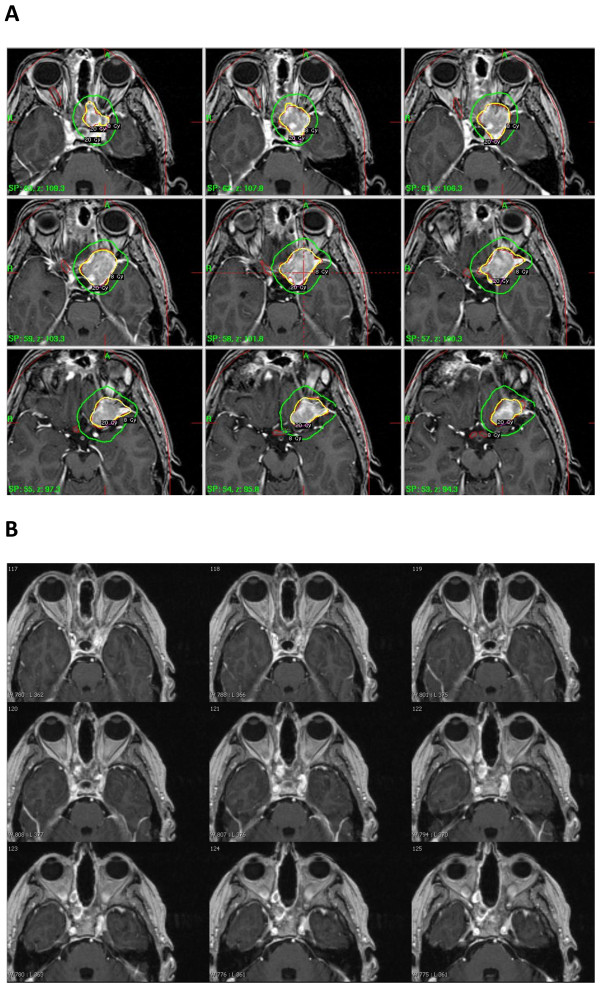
Magnetic resonance images. (A) Axial T1-weighted, contrast-enhanced magnetic resonance images at time of first treatment, showing the left cavernous sinus tumour and treatment isodoses as outlined in the Gamma Knife® planning software. (B) Axial T1-weighted, contrast-enhanced magnetic resonance images after 18 months.
